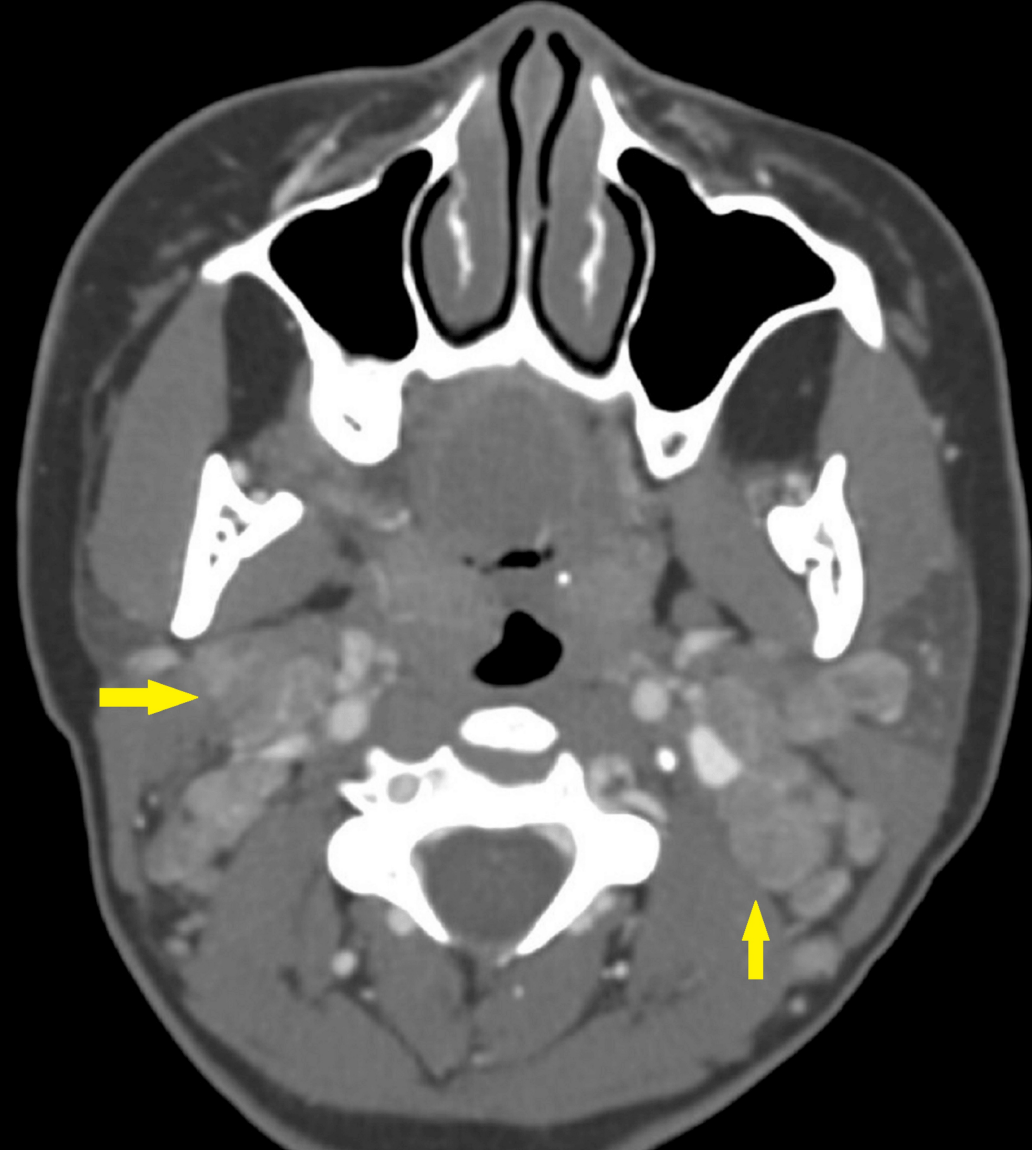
CT neck with contrast, axial view Bilateral enlarged cervical nodes are noted (yellow arrows).
